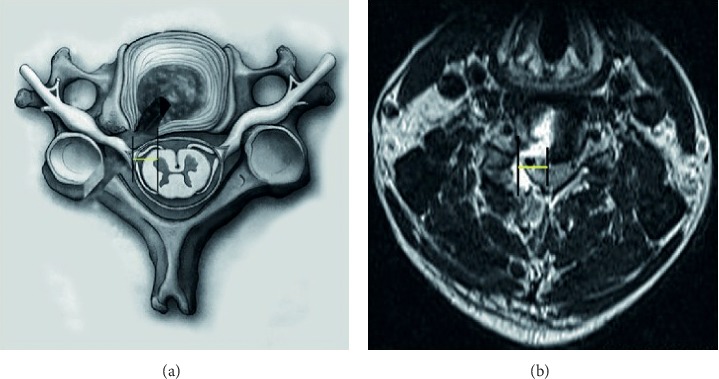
The lateral border of the dural sac and the peak of the herniated disc (DSPHD; red line) and the lateral border of the dural sac and the intersection of the dural sac and the medial border of the herniated disc (DSMHD; blue line) (a) and an MRI image (b). The medial margin of the uncovertebral joint was marked by the red arrow.
